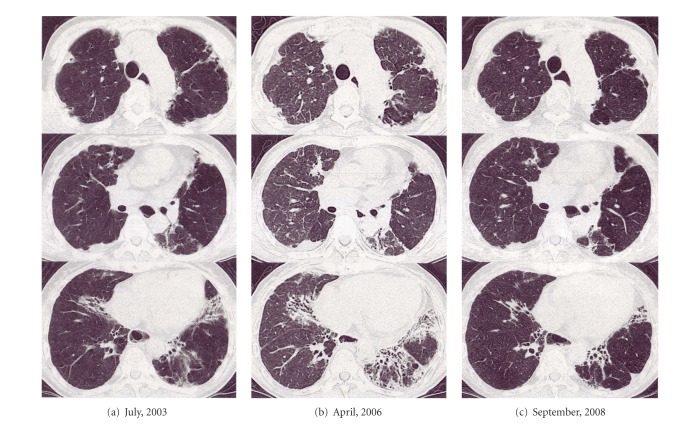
Course of chest HRCT. (a) Consolidations, ground-glass opacities (GGO), and traction bronchiectasis (TBE) were noted on chest HRCT at July, 2003. (b) Consolidations, GGO, and TBE increased on admission in April, 2006, compared with that in April, 2003. (c) Consolidations, GGO, and TBE improved with 10 mg/day of prednisolone and 75 mg/day of azathioprine at September, 2008.
